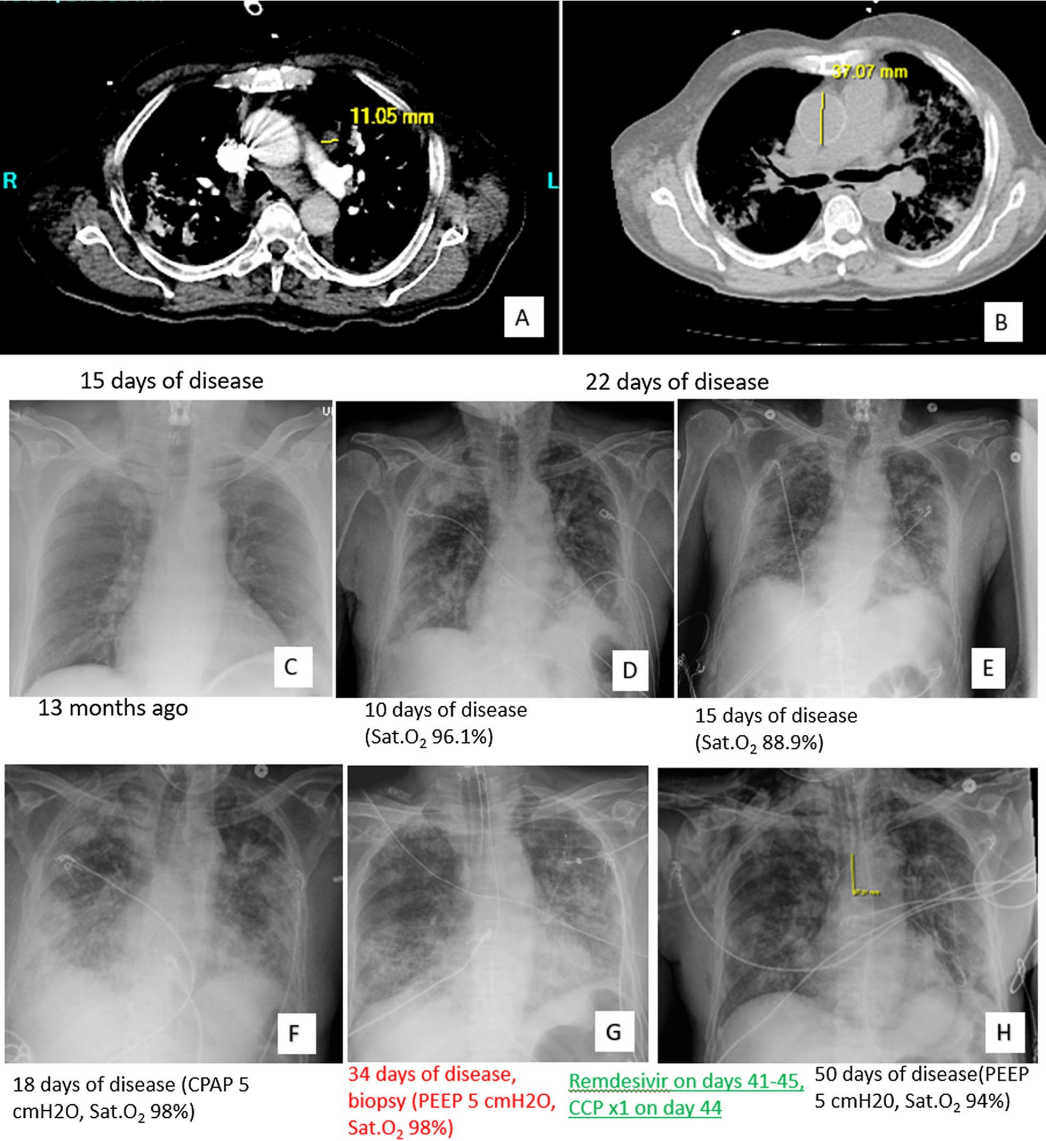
Chest CT and X-ray images. The upper panel (a and b) shows chest CT and the mid and lower panels (c–h) show chest X-ray. The images of chest CT on day 15 (a) and 22 (b) show patchy bilateral lung pulmonary infiltrates with predominant ground-glass components suspicious for pneumonia including COVID19. The new onset disease is mostly peripherally located (d–h) compared to previous asthma exaggeration 13 months ago (c) (mid and lower panel, chest X-ray). The disease was initially stabilized with corticosteroid, antibiotics (including azithromycin) and bronchodilator treatment and patient was not intubated until day 15 (d and e). The disease deteriorated on day 18 (f) and the hypoxemia required ventilation and diagnostic procedure of bronchoalveolar lavage and lung wedge biopsy (g). After the confirmation of COVID19, remdesivir, COVID19 convalescent plasma and continuous corticosteroid as well as bronchodilator led to slightly dissolved lung opacity (h)
